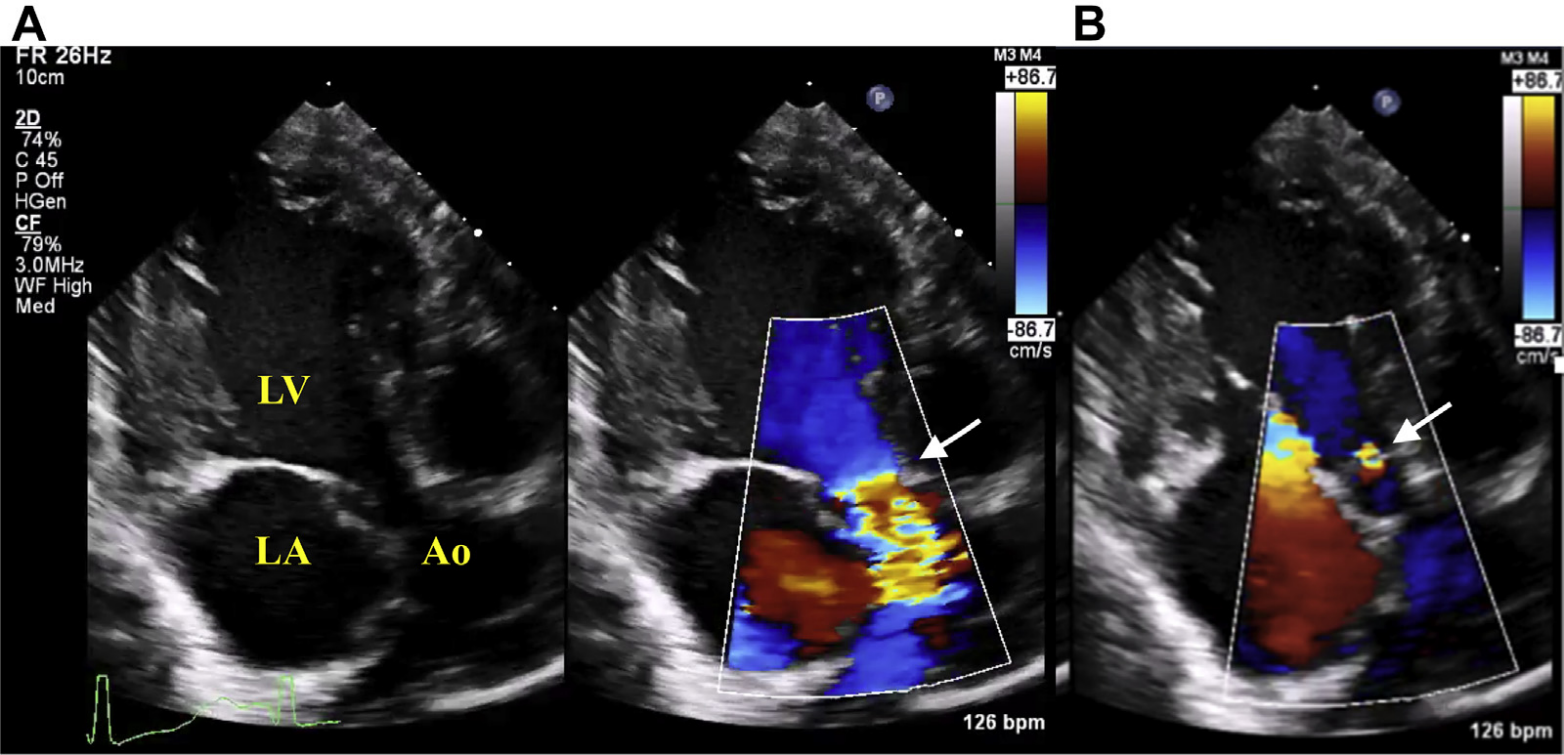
Two-dimensional and color Doppler apical 3-chamber view from TTE showing flow acceleration across the aortic valve in systole (*white arrow*). The peak velocity was 2.7 m/sec with a calculated peak gradient of 29 mm Hg (A) and mild central aortic valve regurgitation in diastole (*white arrow*) with vena contracta of 2.0 mm, jet width/LVOT width of 23%, and jet deceleration rate of 516 msec (B). *Ao*, Aorta; *LA*, left atrium; *LV*, left ventricle.
